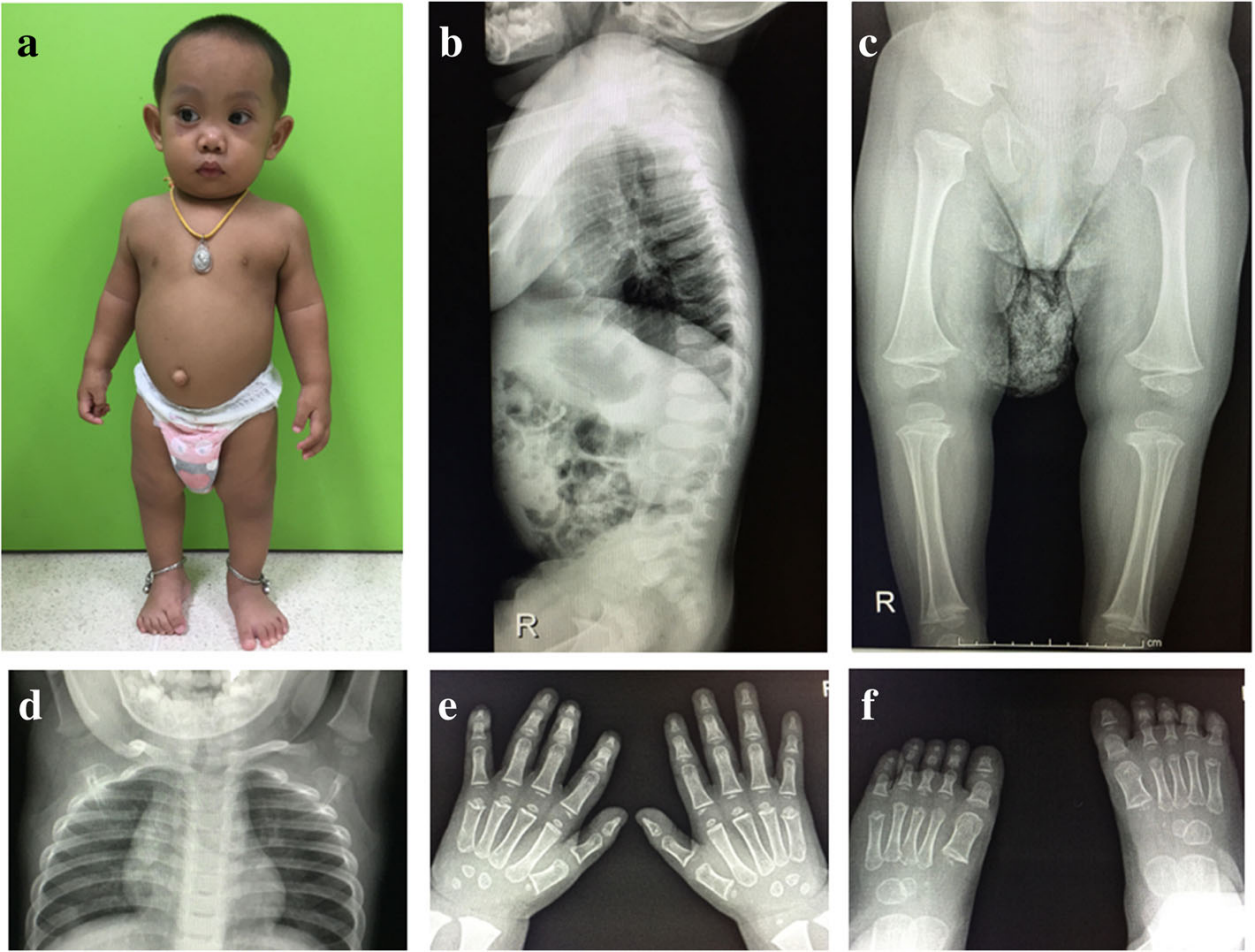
a Photograph of the proband (IV: 3) showing flattened facial profile, micrognathia, short neck, and short trunk. b Whole spine lateral radiograph showing oval-shaped vertebral bodies. c Pelvis, femur, and tibial AP radiograph showing retarded ossification of the femoral heads while other ossification centers are age-appropriate. d Chest radiograph showing age-appropriate ossification centers at humeral heads and greater tuberosities of humeri. e, f Hand and foot AP radiographs are normal
